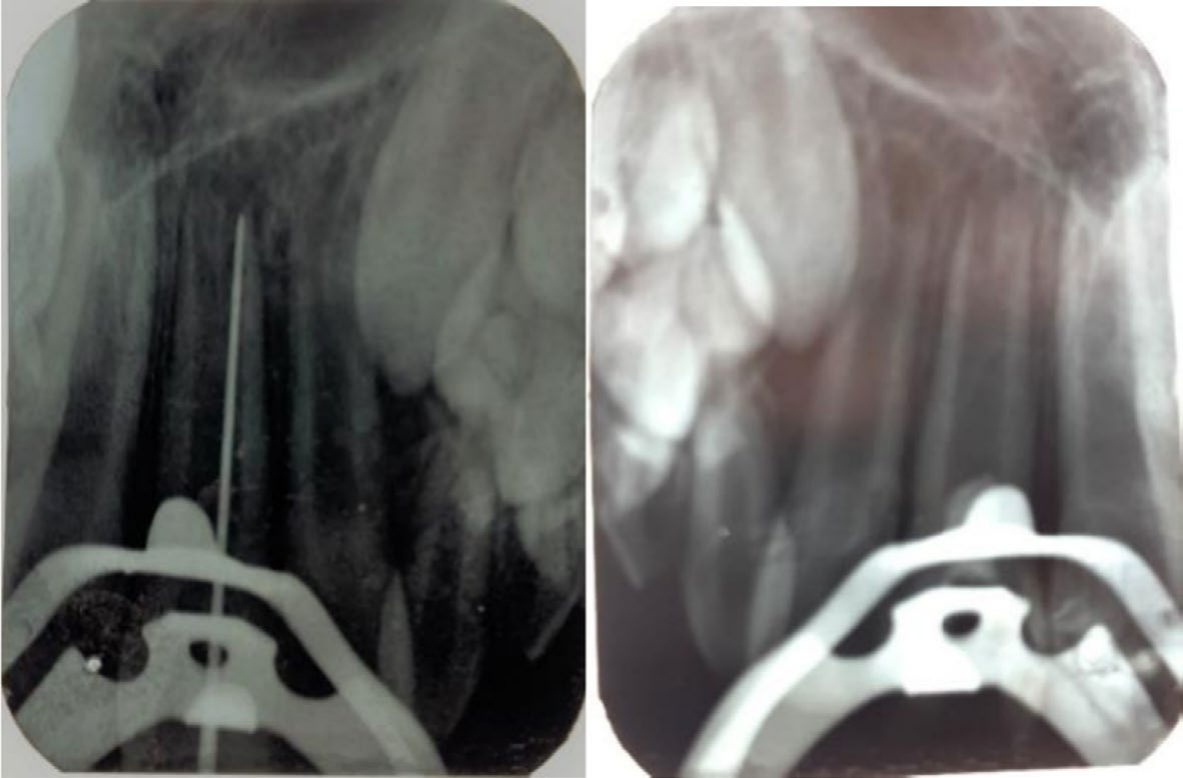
Radiographs of revascularization procedures.
